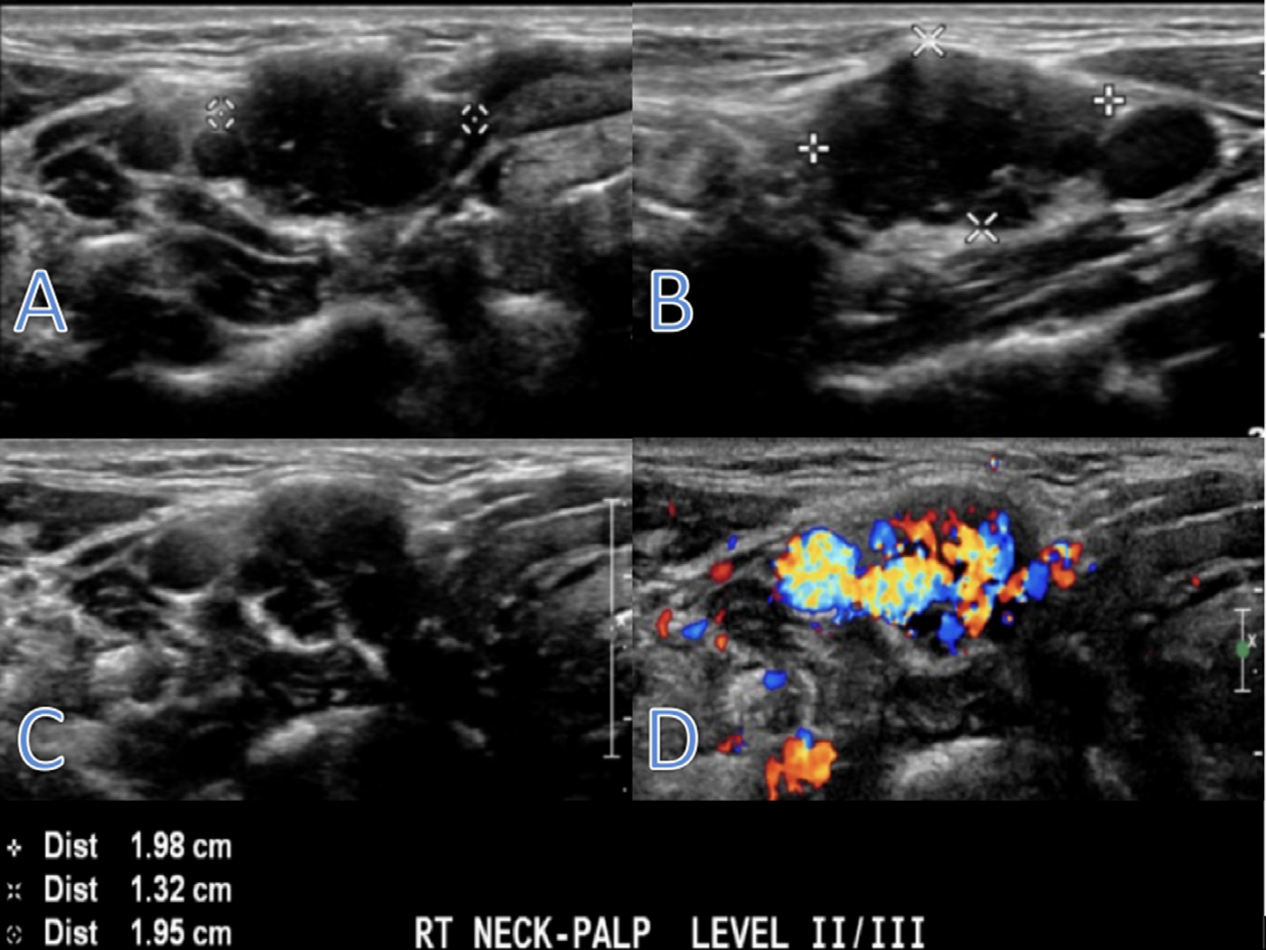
Ultrasound of the neck demonstrating hypoechoic vascular mass lesion just anterior to carotid bifurcation.
